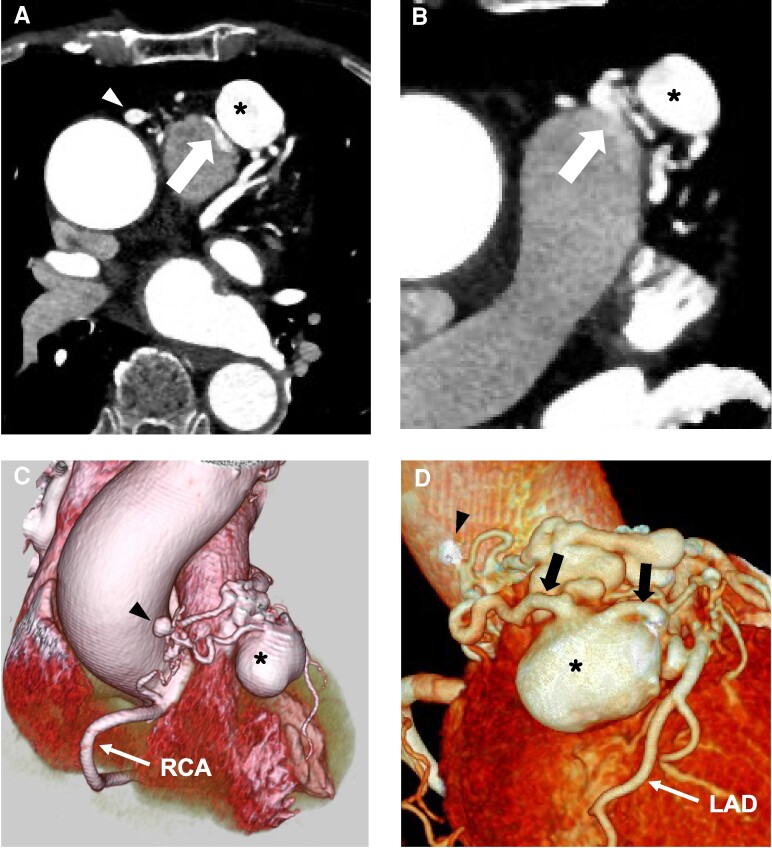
Preoperative multidetector computed tomography. The asterisk indicates a large aneurysm. Computed tomography coronary angiography axial view (*A* and *B*) showing a small aneurysm (arrowhead) and coronary artery-to-pulmonary artery fistula (arrow). Heart surface volume rendering reconstructions (C and *D*) showing a small aneurysm (arrowhead) and large aneurysm (*) with the afferent and efferent vessels (arrows) connecting the left anterior descending artery and right coronary artery.
